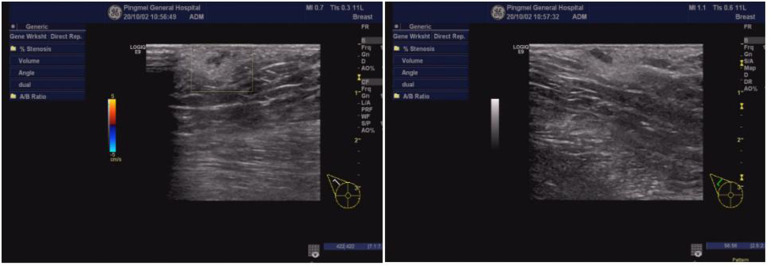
Ultrasound examination of the right armpit. An accessory breast was in the right armpit, at about 0.67  ×  0.49  ×  0.28 cm^3^.
